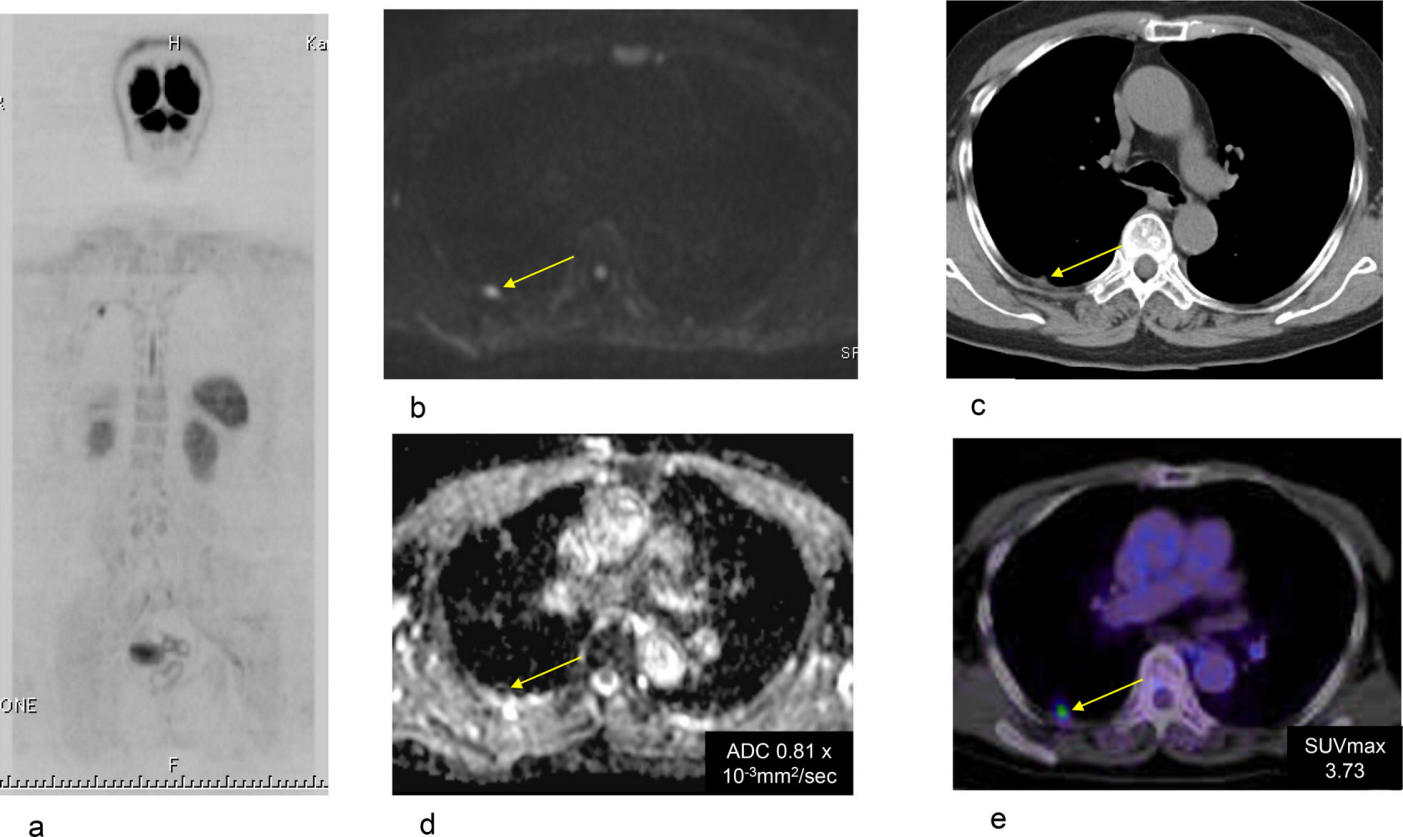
Case 3. Adenocarcinoma, pT2aN0M0. (a, b) Pleural metastasis was present in diffusion‐weighted whole‐body imaging with background suppression (DWIBS), (c) CT and (e) fluorodeoxyglucose positron emission tomography (FDG‐PET/CT). The apparent diffusion coefficient (ADC) of the pleural metastasis in the ADC map was 0.81 × 10^−3^ mm^2^/s (d). The SUVmax of the pleural metastasis in FDG‐PET/CT was 3.73 (e). A tiny metastatic lesion was detected on DWIBS
